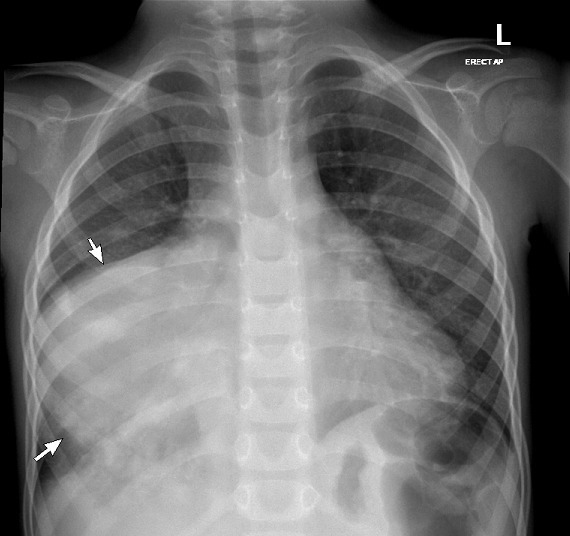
Chest X-ray showing a large mass in the right lower hemithorax with broad mediastinal attachment (arrows). Thickening of the right para-tracheal stripe that could be related to paratracheal lymphadenopathy.
